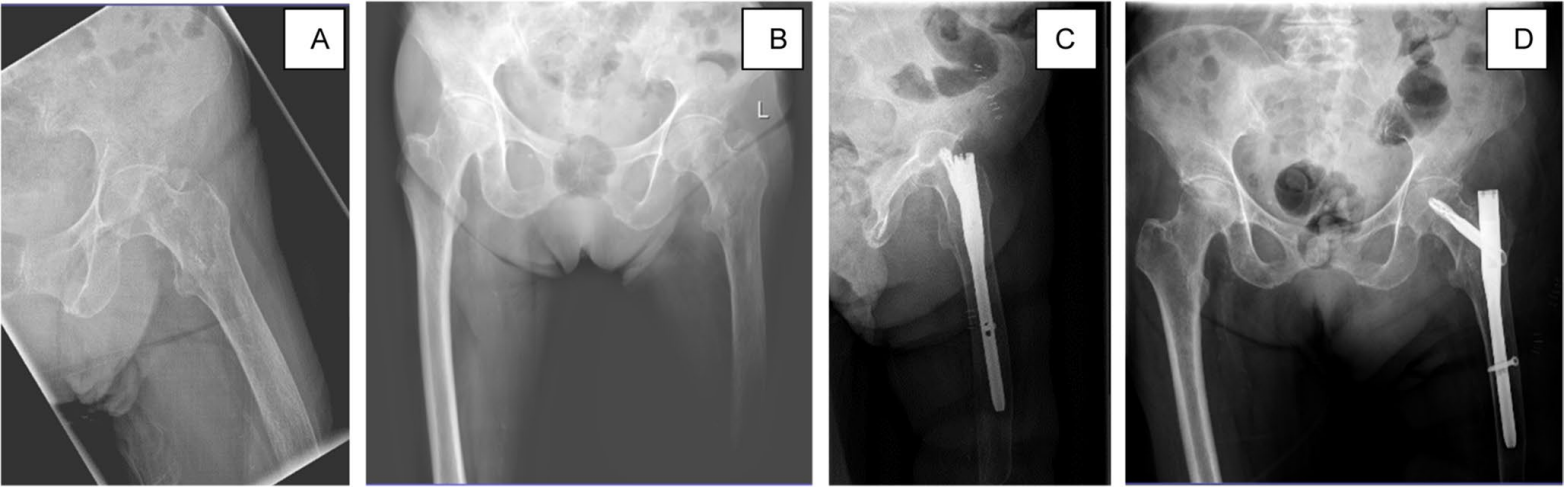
Preoperative radiographs showing a left pertrochanteric femur fracture (A, B). Postoperative radiographs showing position of the cephalomedullary nail (C and D)
